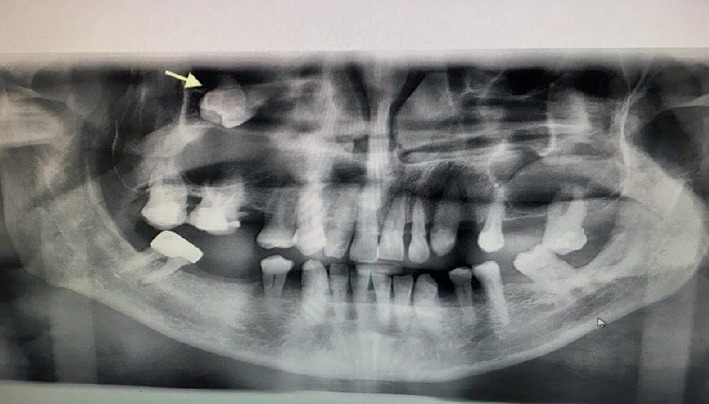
Preoperative dental panoramic radiograph showing ectopic tooth (arrow) in the right maxillary sinus.
